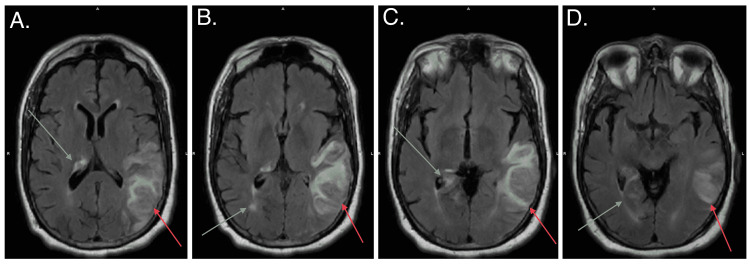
Brain MRI showing subependymal signal abnormalities with enhancement in the right periventricular white matter, thalamus, hippocampal tail, and bilateral occipital lobes (grey arrows) and suspicious left neoplasm (red arrows).
